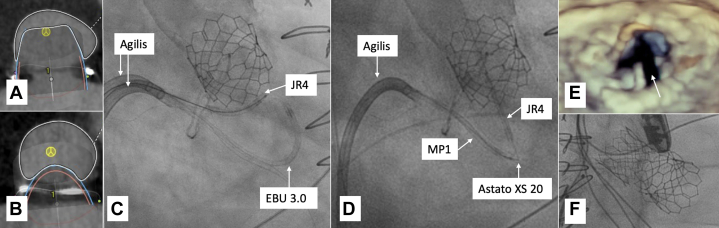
Imaging for Case 3 (A) CTA of neo-LVOT 249 mm^2^ without LAMPOON. (B) CTA rendering of neo-LVOT 426 mm^2^ with LAMPOON. (C) Fluoroscopy of antegrade LAMPOON. (D) Fluoroscopy of tip-to-base LAMPOON. (E) Three-dimensional TEE after LAMPOON showing anterior mitral leaflet laceration (yellow arrow). (F) Fluoroscopy showing valve-in-ring TMVR with 29-mm Edwards S3 and previously implanted TAVR with 26-mm Edwards Sapien S3. TAVR = transcatheter aortic valve replacement; TMVR = transcatheter mitral valve replacement; other abbreviations as in Figure 1.
